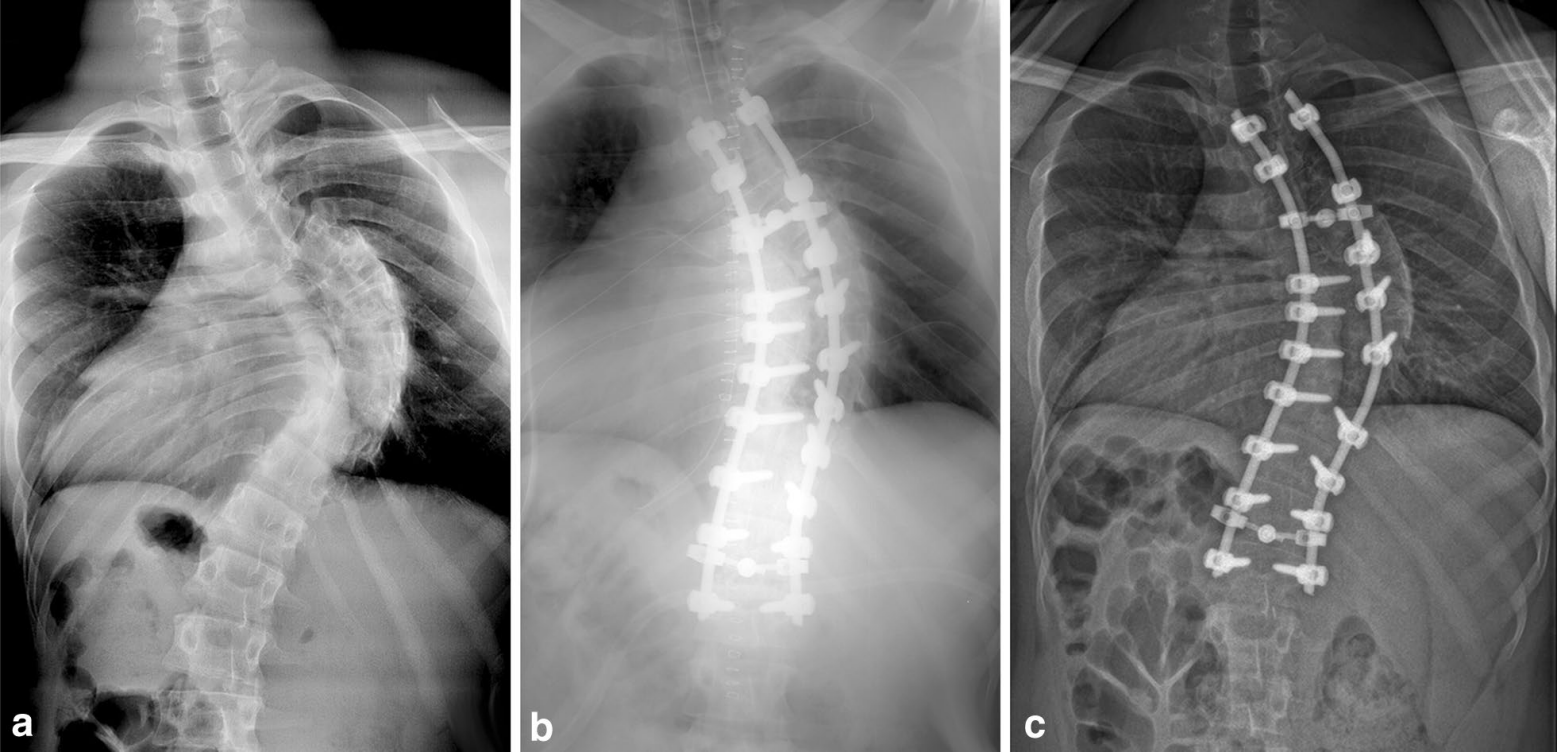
a Preoperative frontal X-ray of the right 95° thoracic scoliosis. b Postoperative frontal X-ray after index procedure. c Postoperative frontal X-ray after revision procedure and screw removal
